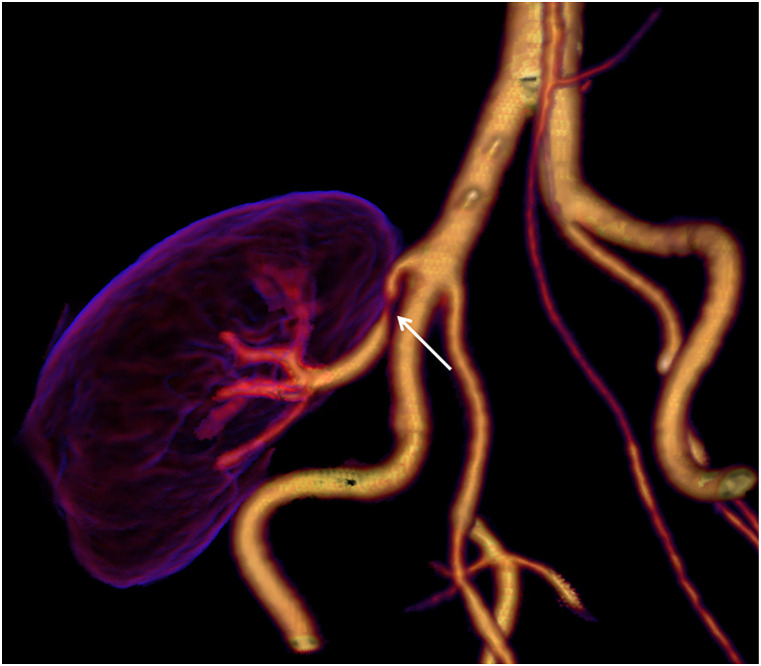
Significant stenosis of the renal artery (arrow). After CT examination, the patient was referred for acute percutaneous transluminal angioplasty (PTA), confirming the renal CTA finding.
